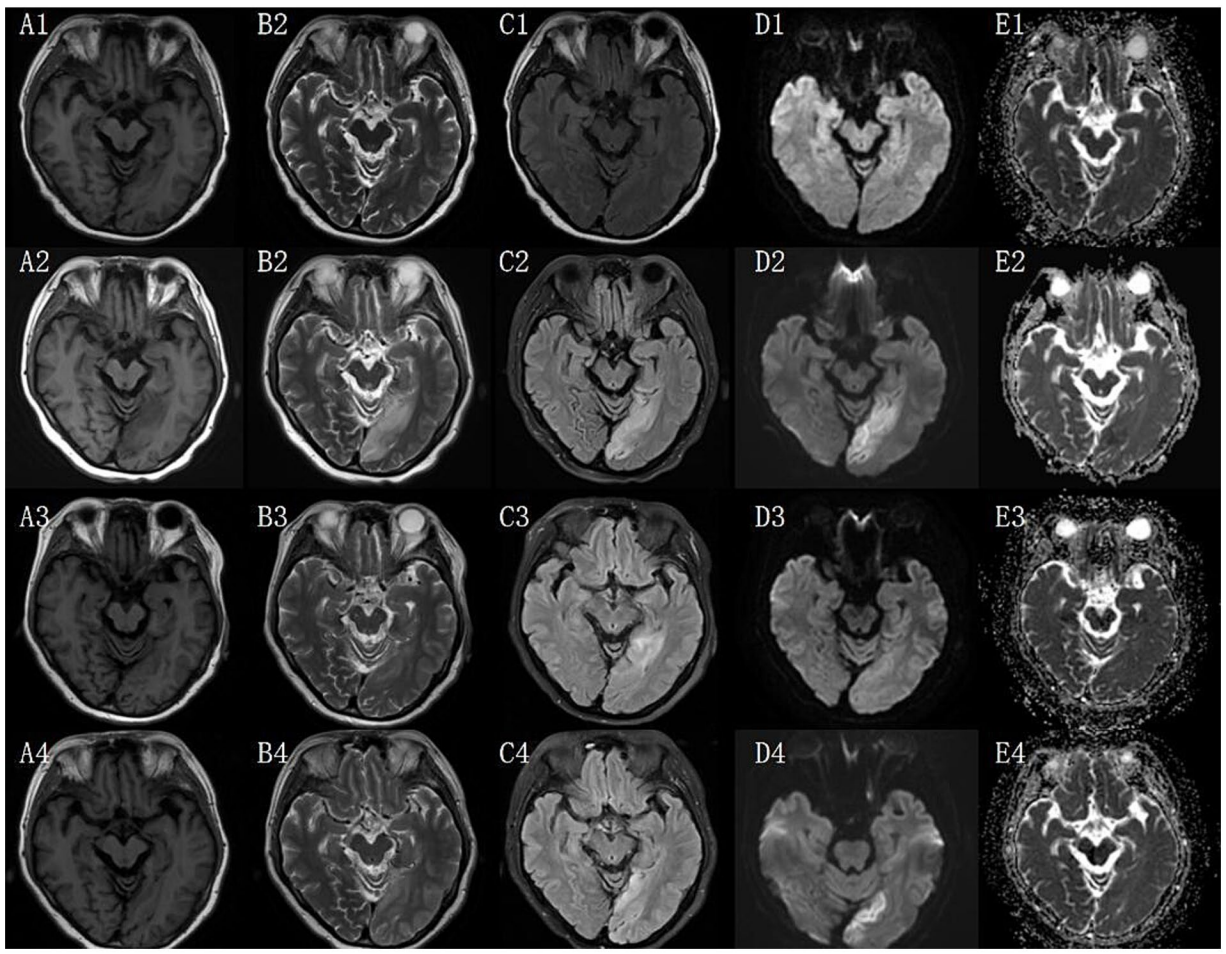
Cranial brain scan + DWI + contrast enhancement (3 T). T1, T1-weighted imaging; T2, T2-weighted imaging; FLAIR, fluid-attenuated inversion recovery; DWI, diffusion-weighted imaging; (A1–E1) 2021/11/21 cranial MRI; (A2–E2) 2024/07/24 cranial MRI; (A3–E3) 2024/08/08 cranial MRI; (A4–E4) 2025/01/10 cranial MRI.
